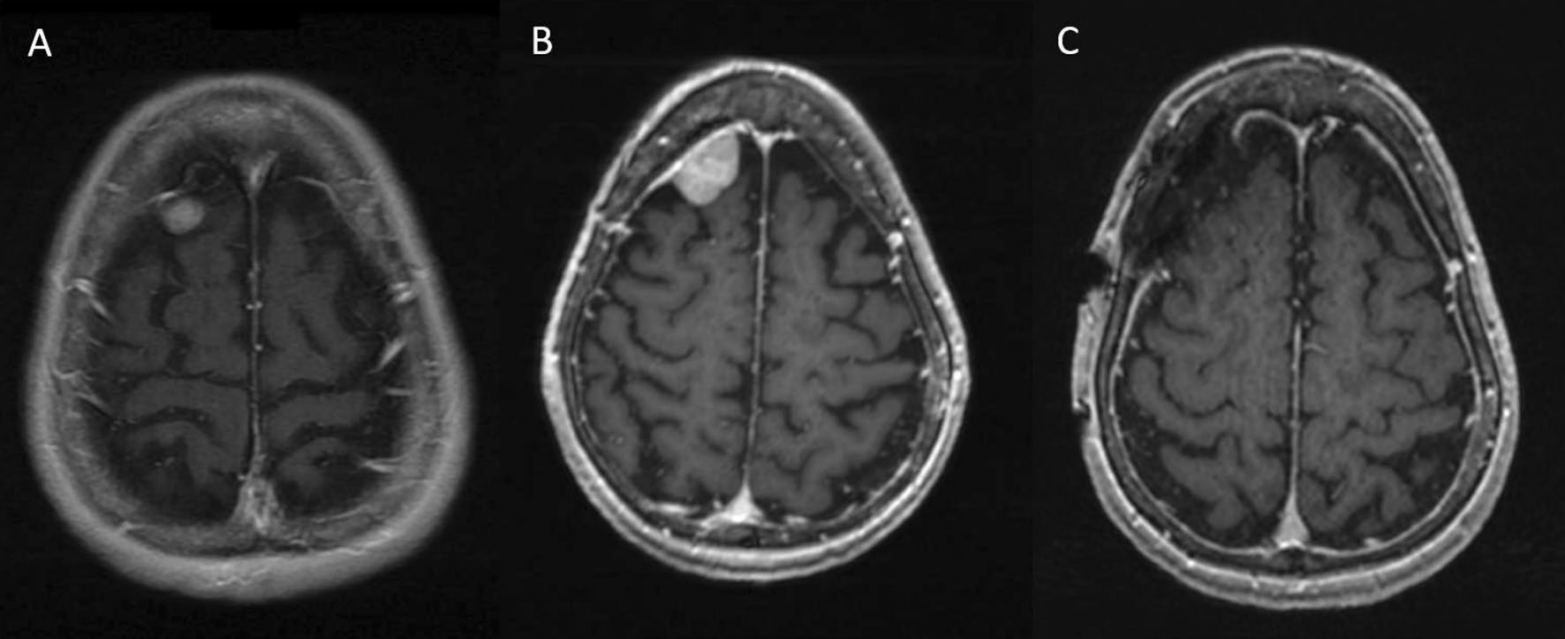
78-year-old female who initially presented with symptoms of dizziness, and T1 MRI with contrast demonstrated an extra-axial lesion measuring 1.4 × 0.8 × 0.8 cm (A). The lesion was presumed to be a meningioma and no resection was recommended at the time. Two years later, serial imaging demonstrated an increase in size of the mass (B). The patient underwent gross total resection (C), and pathology confirmed a WHO grade 2, atypical meningioma. Genomic report demonstrated an aggressive profile, with an NF2 somatic driver mutation and multiple copy number variants including chr1p deletion, chr6 deletion, chr18 deletion, chr19p deletion, and chr22 (NF2) deletion. She completed a course of adjuvant radiation therapy
